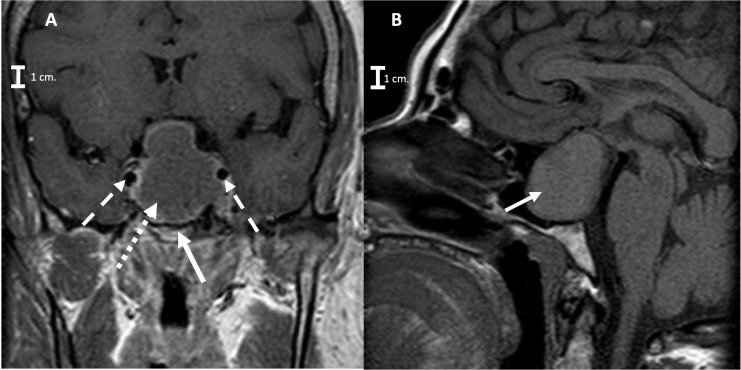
(A) Preoperative coronal Brain MRI T1 signals respectively, showing the pituitary macroadenoma (arrow with short dashed line) with suprasellar extension and selllar floor erosion (arrow with continues line), without encasement of the carotids (arrows with long dashed line pointing both carotids). (B) Sagittal brain MRI T1 signals respectively (arrow with continues line is pointing to the Macroadenoma).
